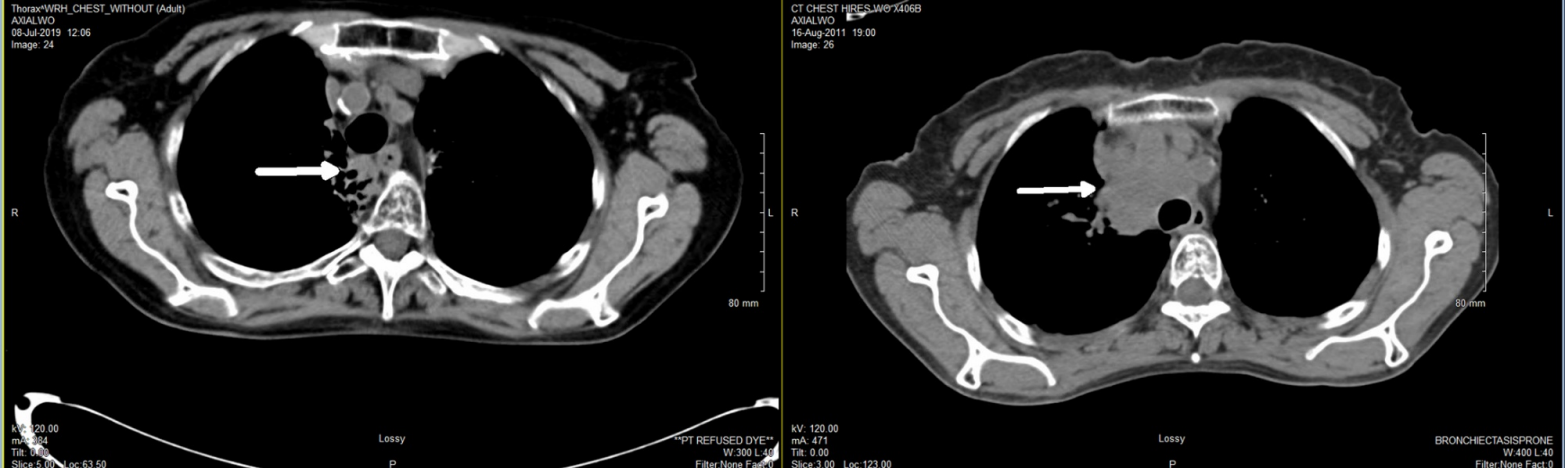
CT in July 2019 showing no signs of recurrence with stable post-radiation changes in the right lung (left) comparing to the original CT in 2011 (right)
